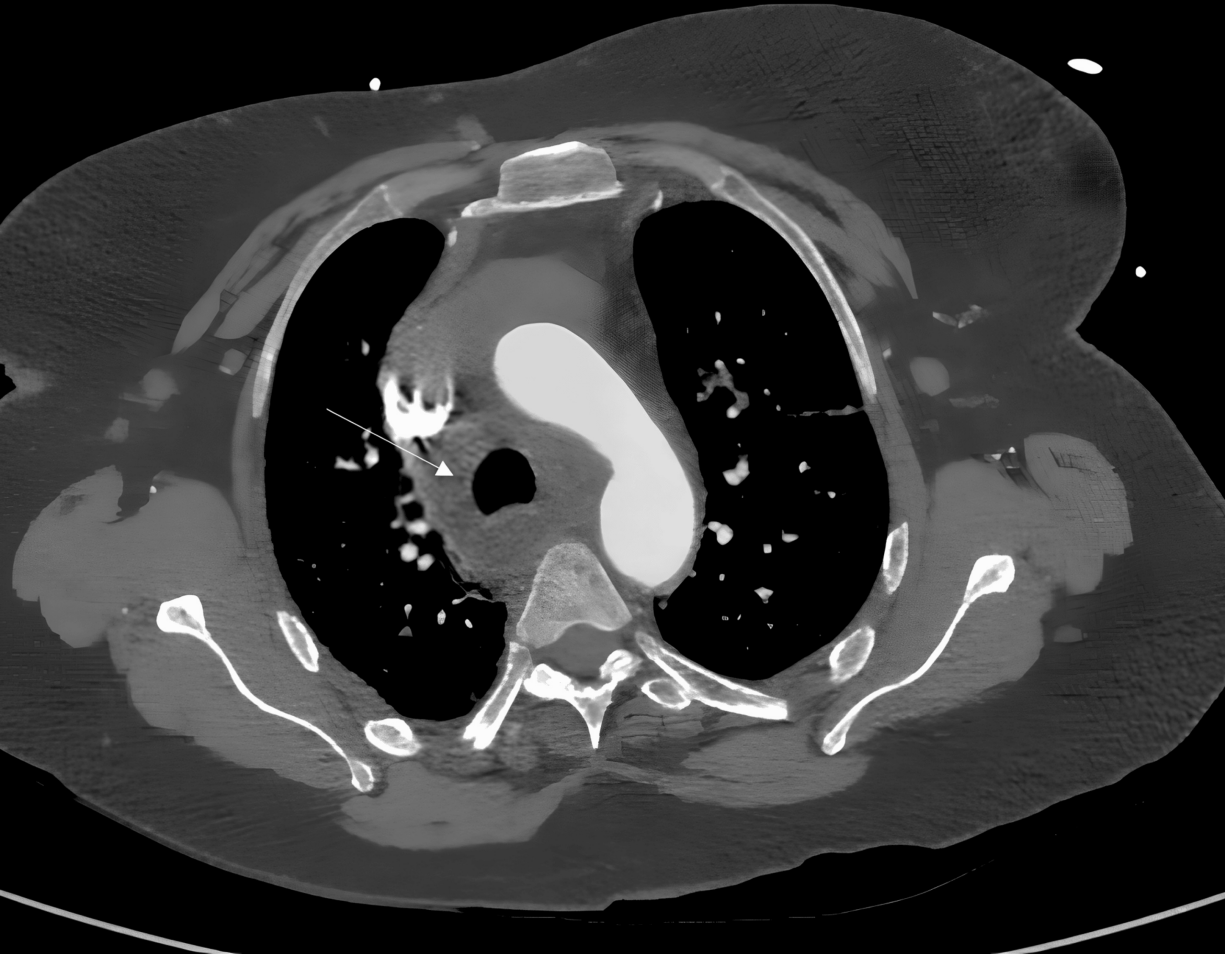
CT pulmonary angiogram - mediastinal window showing an enlarged right paratracheal lymph node (white arrow)
